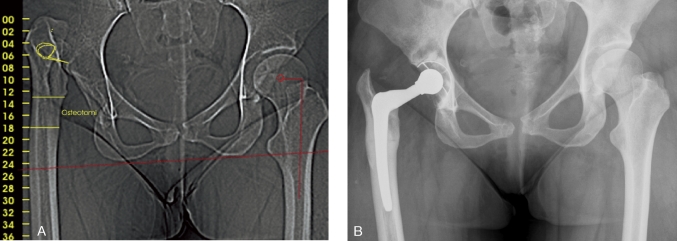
A. Scanogram of the right femur in a case of femoral head necrosis with high dislocation of the hip. B. Radiograph after subtrochanteric resection osteotomy and insertion of the custom stem. The osteotomy has healed.
